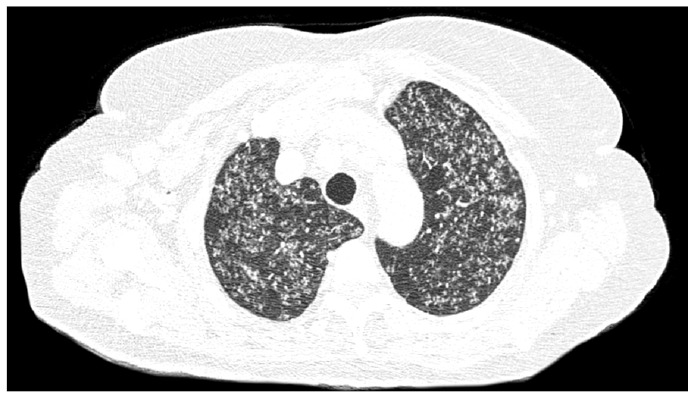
Chest computed tomography; lung window. Diffuse miliary nodules.
